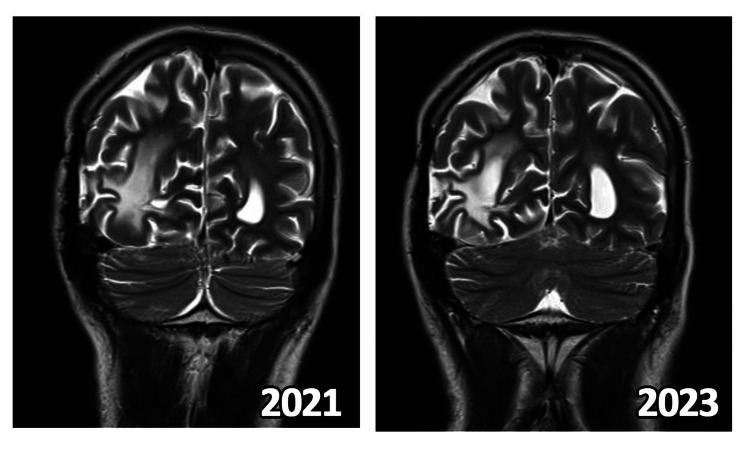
MRI of Patient 2 after initiation of lomustine and most recent imaging follow up
